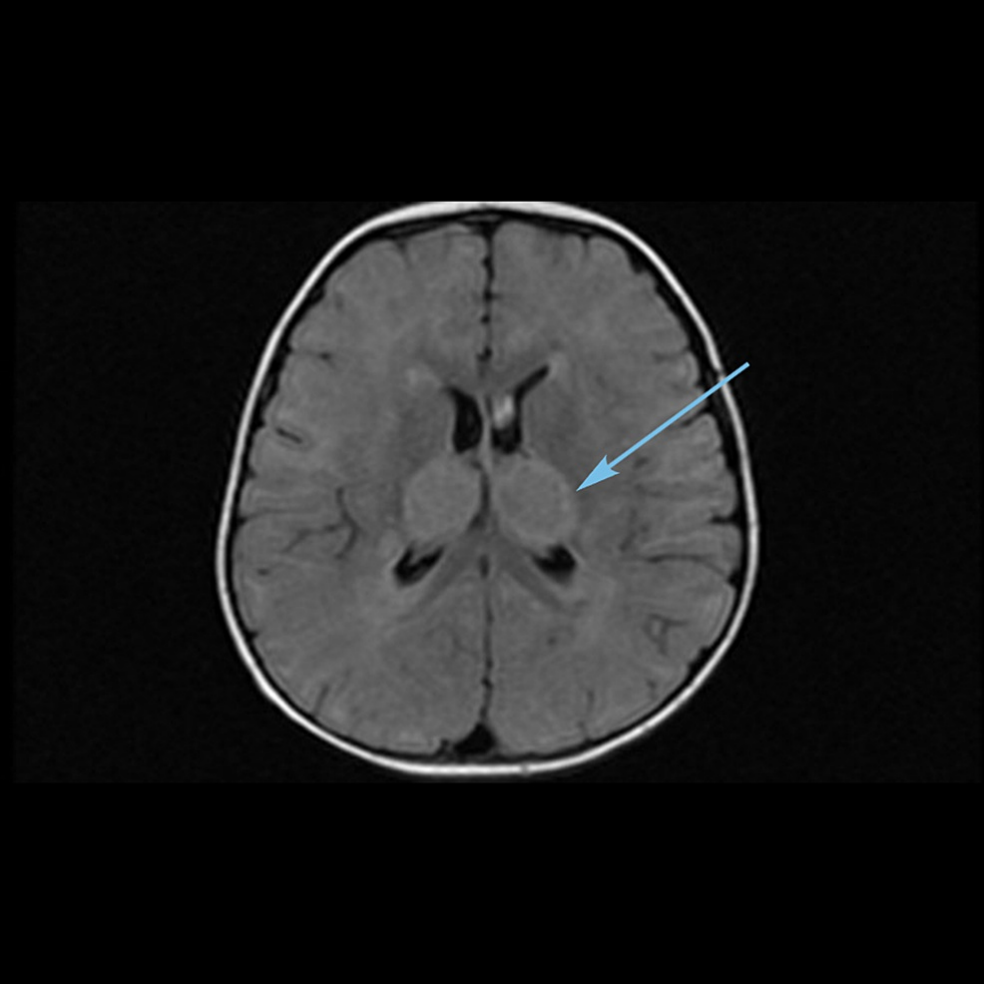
Repeat MRI brain axial image showing hemorrhagic changes in bilateral thalami (arrow).
